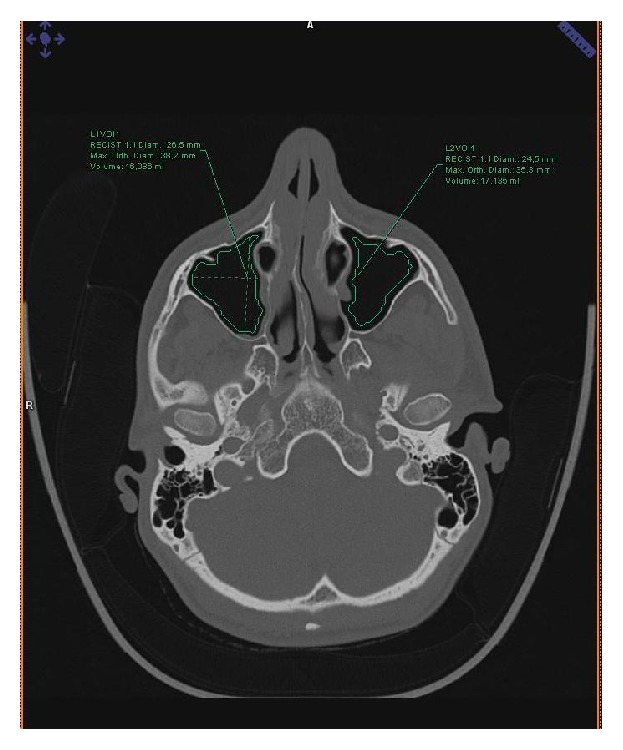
Examples of MSW and MSL measurements on the CT image.
